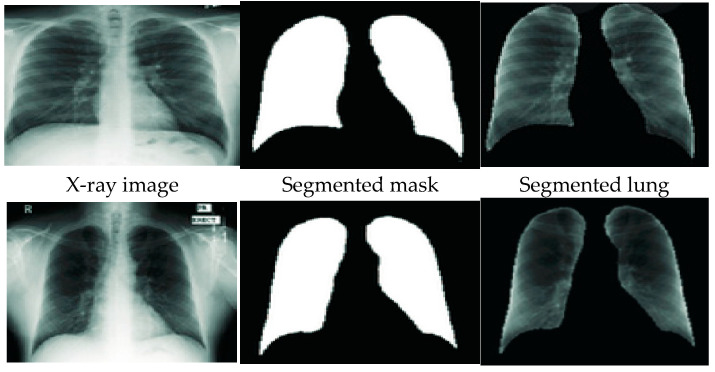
Sample of segmented mask and segmented lung from the base X-ray images.
